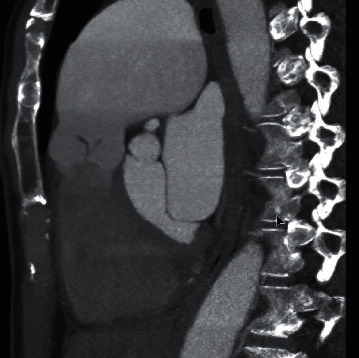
Cardiac CT sagittal section with PAA and pulmonic valve anatomy.
